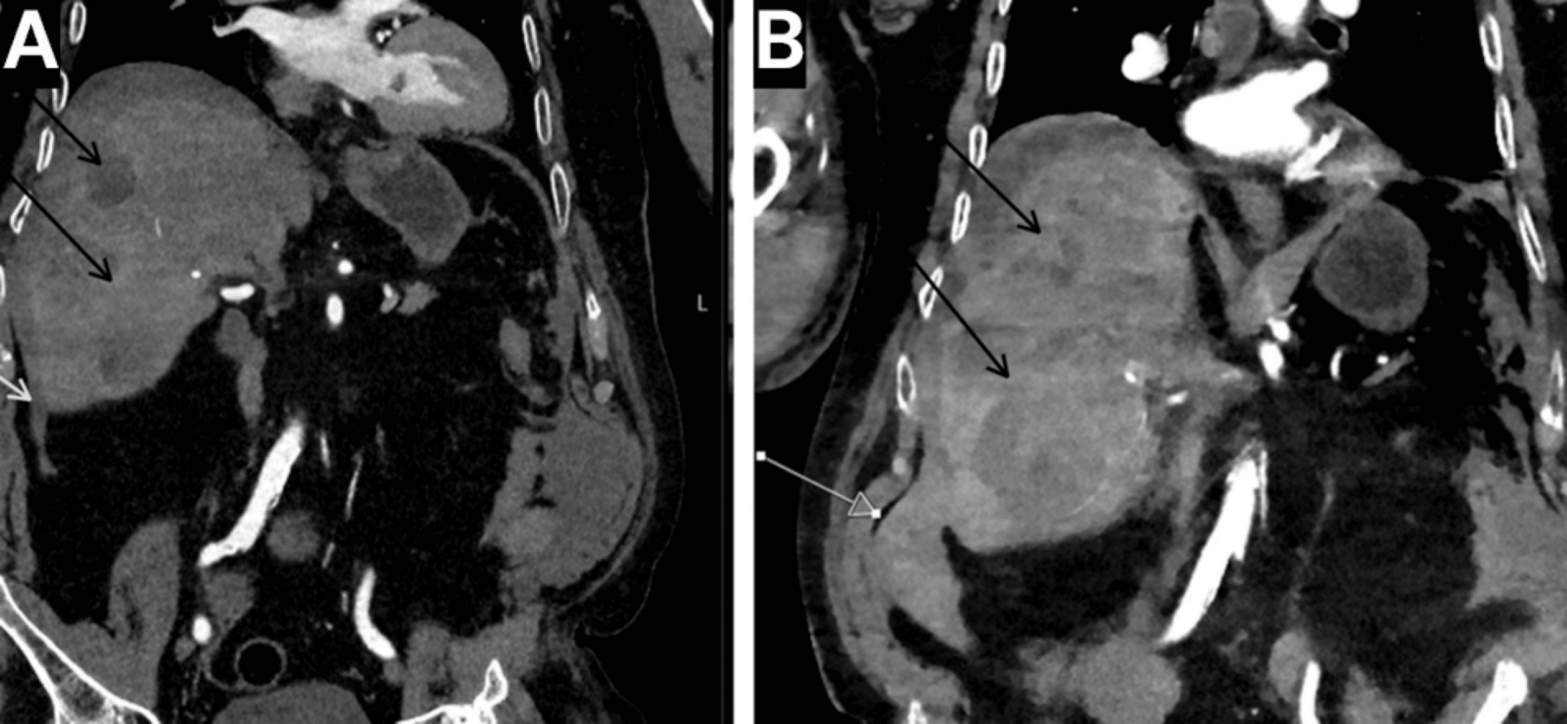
Interval progression on follow-up CT mesenteric angiogram compared with initial CT aorta (A) Contrast-enhanced CT aorta showing subcapsular hepatic hematoma (white arrow) and hepatic metastases (black arrows). (B) Follow-up CT mesenteric angiogram 10 days later, demonstrating expansion of the subcapsular hepatic hematoma (white arrow) and interval progression of hepatic metastases (black arrows).
